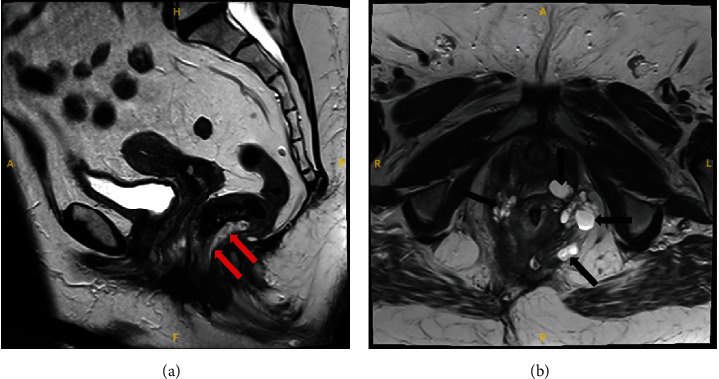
Pelvic MRI 13 months later with a sagittal T2 weighted image (a) and an axial T2 weighted image (b) demonstrated a T2 hyperintense mass within the posterior wall of the low rectum (red arrow) and multiple additional T2 hyperintense perirectal masses (black arrow).
